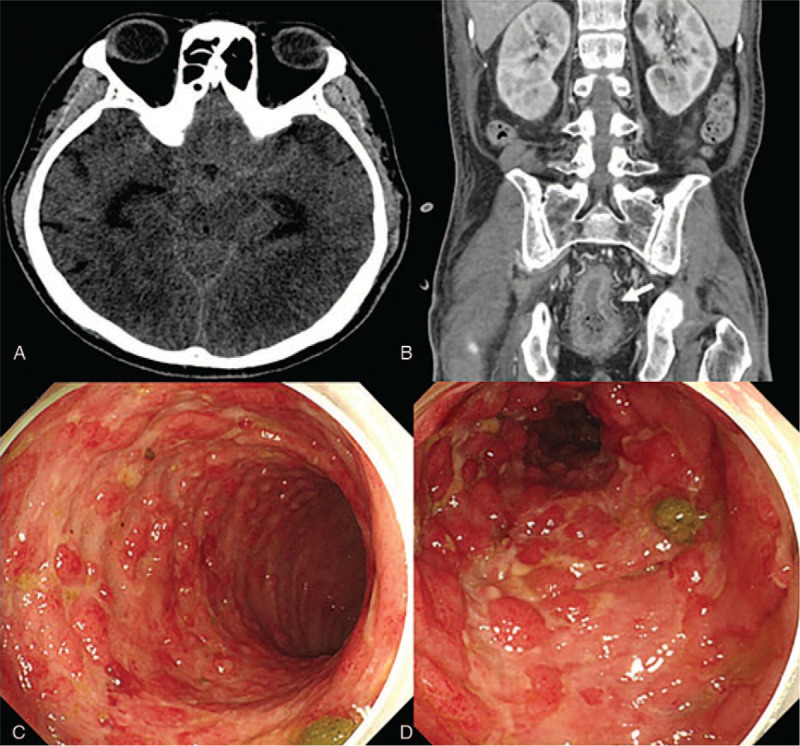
Case 2. A: SAH with no aneurysm sac. B: APCT revealed edematous wall thickening in distal sigmoid colon and rectum, suggesting proctocolitis (white arrow). C and D: Colonoscopy showed diffuse ulcerative lesions with mucosal friability. APCT = abdomen/pelvis computed tomography, SAH = subarachnoid haemorrhage.
